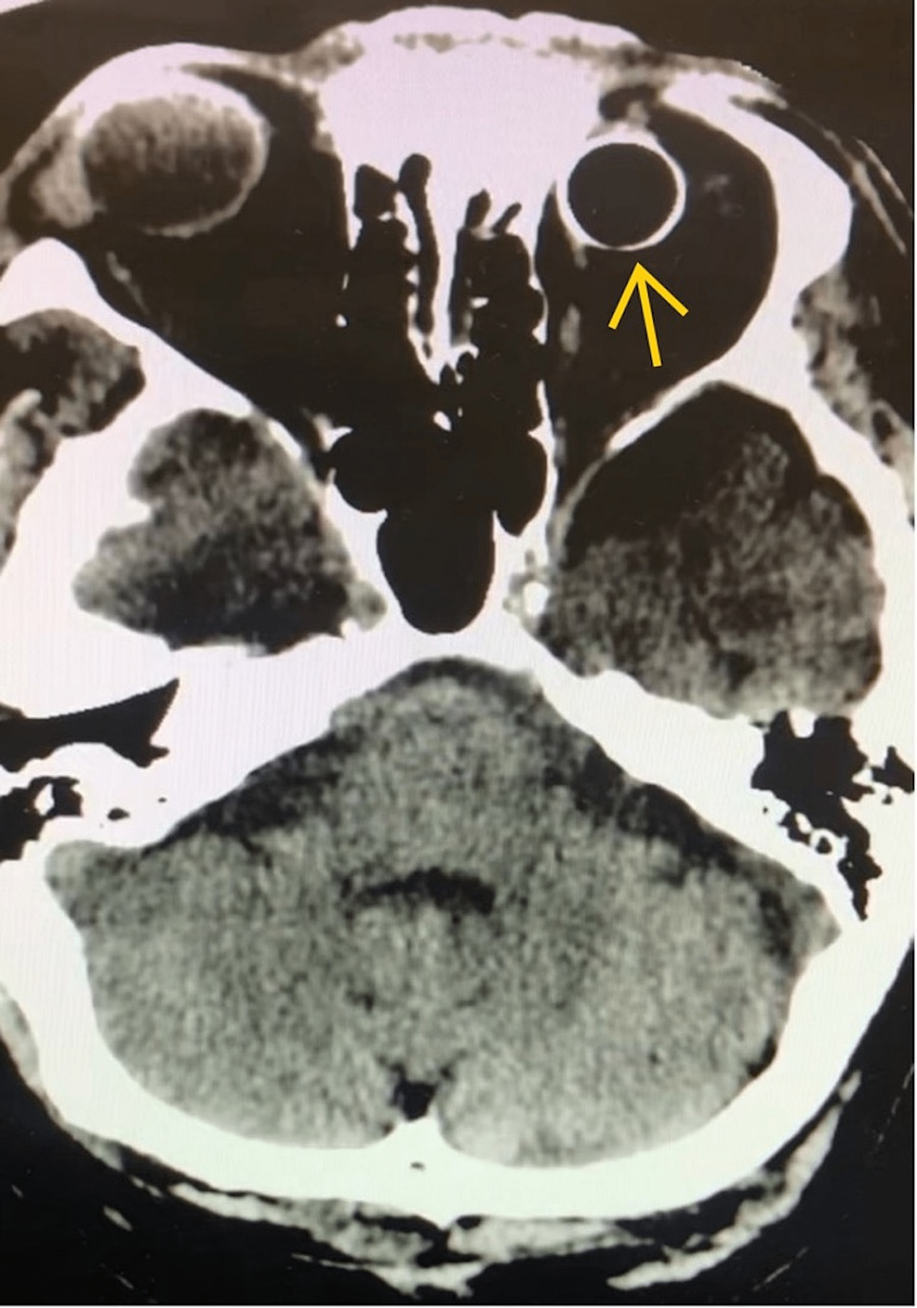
CT imaging of our patient, read by neuroradiology as having Hounsfield scale analysis consistent with a metal shell, showing similarities with imaging from a case report of a 1960s implant having a metal shell.
